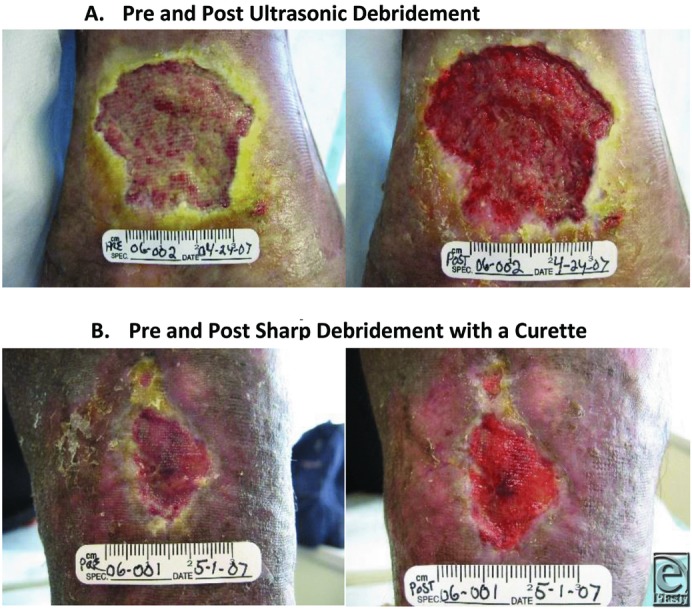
(*a*) Pre- and post–ultrasonic debridement. (*b*) Pre- and post–sharp debridement with a curette.
